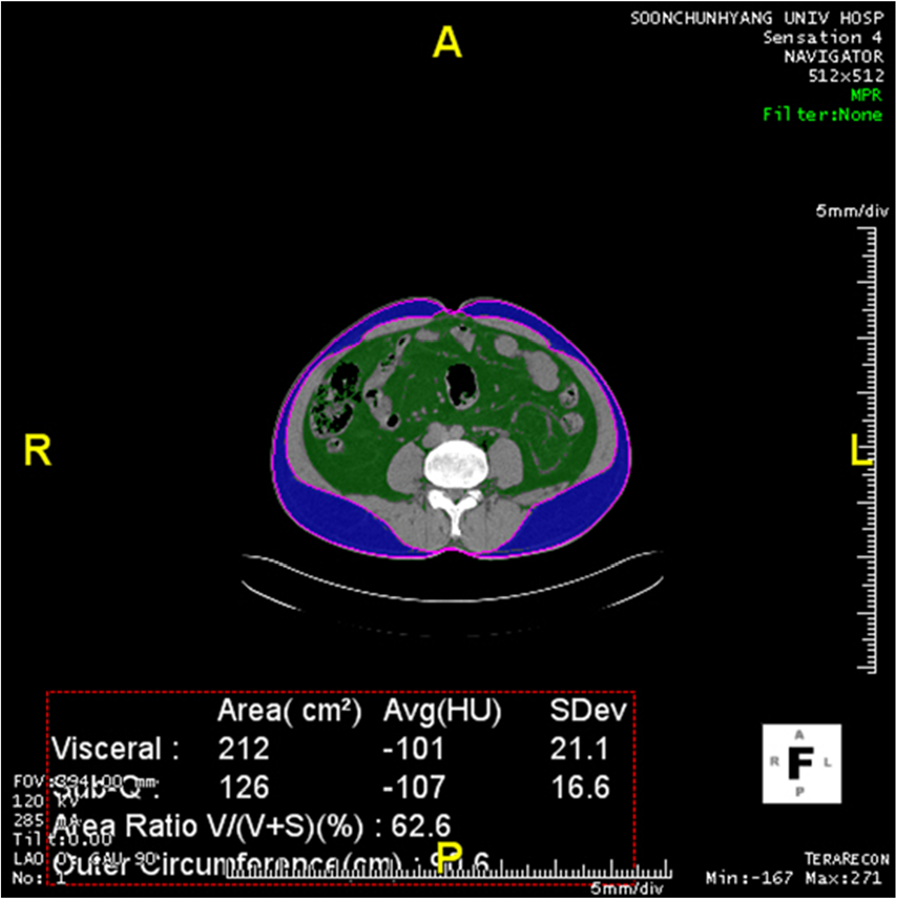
CT fat delineation in a male with visceral fat obesity without obesity whose visceral fat was 212 cm
